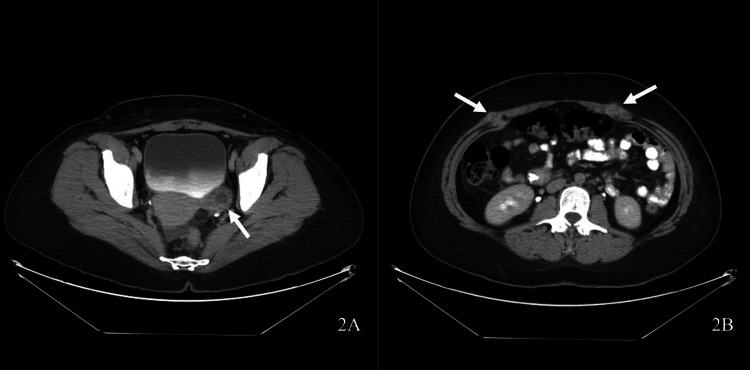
Axial abdominal CT scan demonstrating (A) a complex left adnexal mass and (B) trocar implants consistent with port site metastasis (arrows).
